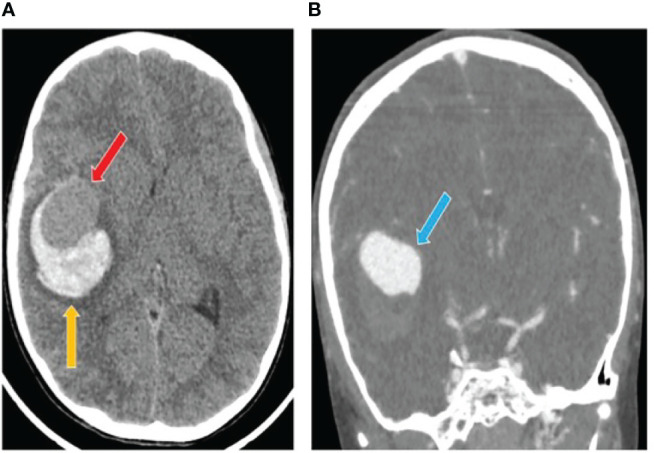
Computed tomography (CT) scan after intracranial aneurysm rupture. (A) The initial head CT showed a thin subarachnoid hemorrhage and a large intra-parenchymal hemorrhage (yellow arrow) including an iso-dense region (red arrow). (B) The angio-TC showed a cerebral aneurysm originating from the right middle cerebral artery (blue arrow).
